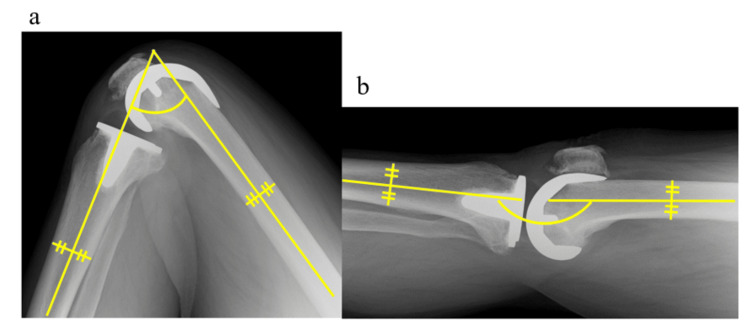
A representative radiographic image (sagittal view) of the knee joint All patients were imaged in full extension and full flexion of the knee joint in the supine position on the sagittal radiographic view. The angle between the line passing through the center of the tibia and that of the femur was measured. (a) shows the maximum flexion angle and (b) shows the maximum extension angle. For this paper, (b) is represented by an angle of 180 minus (b).
